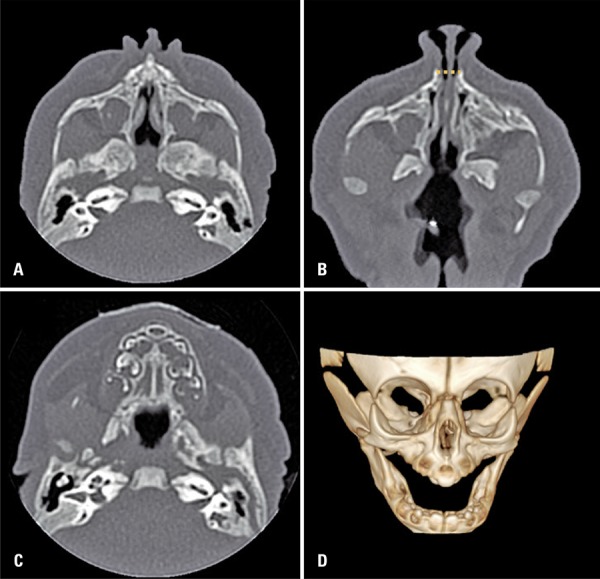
Axial computed tomography scan sections of paranasal sinuses. (A) Open choanae, no atresia. (B) Bilateral and symmetrical thickening and medialization of the anterior aspect of the maxilla, which determines extreme narrowing of pyriform aperture (dotted line, measuring about 0.6cm, normal value = 1.1cm). The image is compatible with congenital pyriform aperture stenosis. (C) Single central megaincisor in median maxillary position and hard palate with triangular configuration, both frequent findings in congenital pyriform aperture stenosis. (D) Three-dimension reconstruction shows narrowing of pyriform aperture and single central megaincisor
